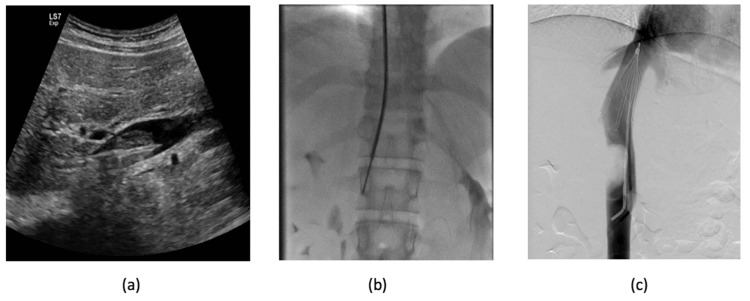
Intravenous biopsy of the thrombus Trans-jugular biopsy of the caval mass/thrombus under direct transabdominal ultrasound (a) and fluoroscopic guidance (b) with insertion of a suprarenal IVC filter (asterisk) (c).
